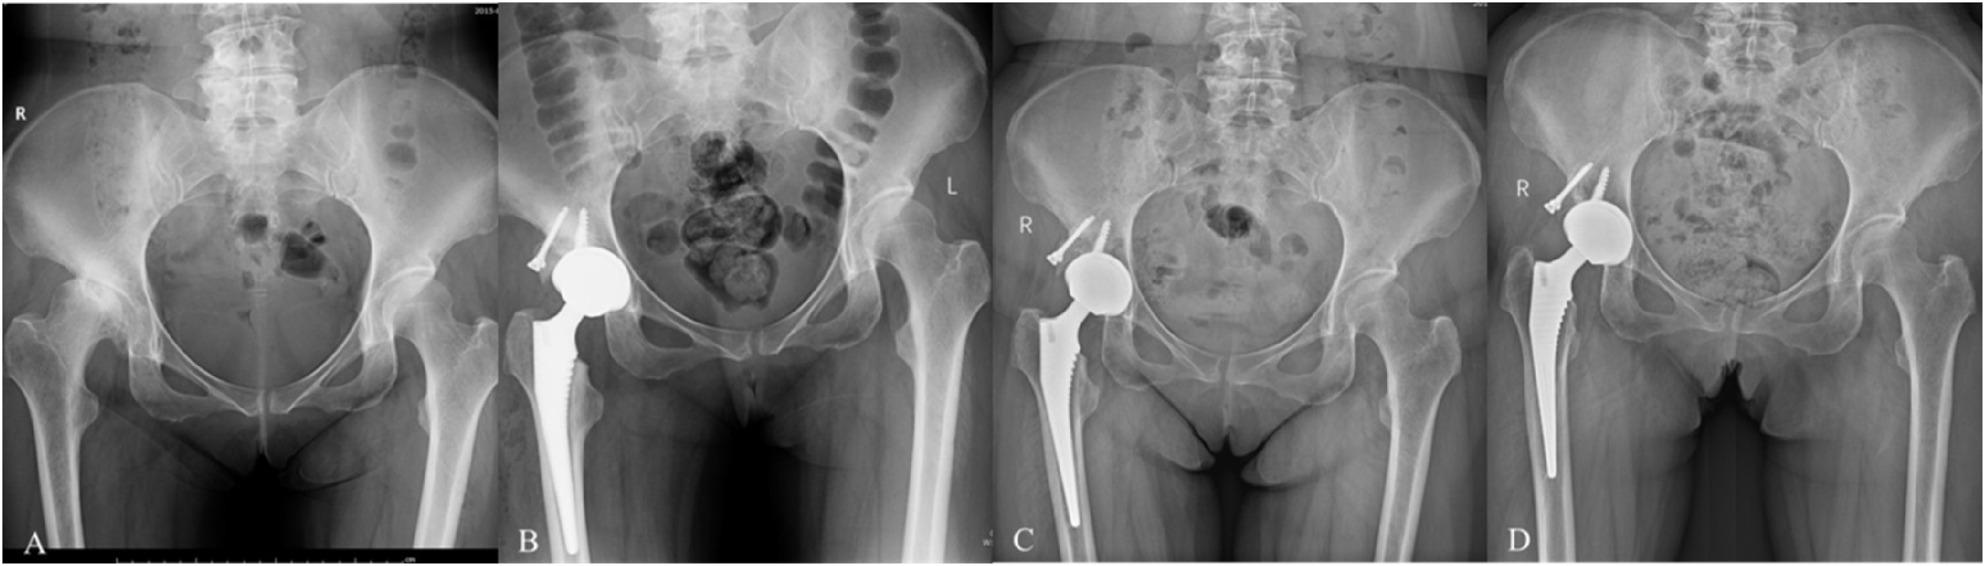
A patient in the early fifties with right-sided DDH, Crowe type II. A Preoperative pelvic radiograph shows right acetabular dysplasia with roof defect (CE angle = 10°). B Postoperative X-ray demonstrates biological acetabular reconstruction with autologous femoral head structural bone graft, achieving sufficient acetabular component coverage (graft coverage = 23%, acetabular component coverage = 98%). C One-year postoperative X-ray shows good graft healing and satisfactory osseointegration at the prosthesis-bone interface (CE angle = 25°). D Final follow-up (10 years postoperatively) X-ray reveals stable prosthesis-bone interface without signs of loosening, complete integration of graft and host bone, and maintained graft morphology without significant volume reduction or resorption. LLD improved from 10 mm to 0 mmFig. 4 Fig4:
